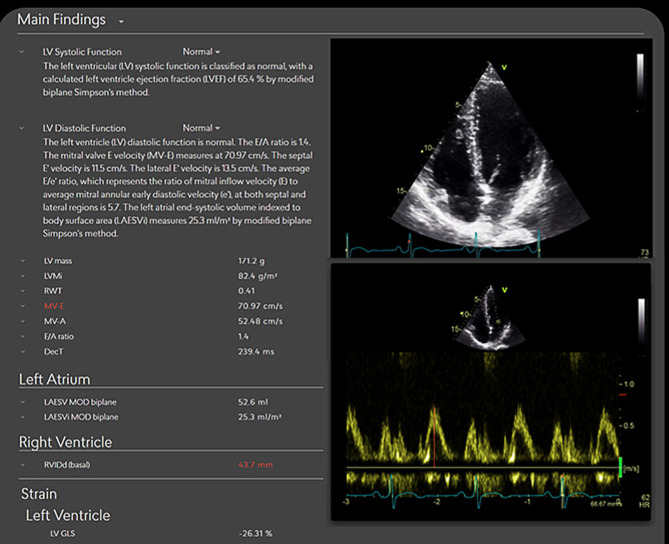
AI-generated echocardiography report using Us2.AI.* Example of an automated report displaying measurements for systolic and diastolic function, left atrial volume, right ventricular size, and strain, with editable contours and Doppler tracings. All outputs are presented in a guideline-aligned format, allowing clinician review and adjustment
